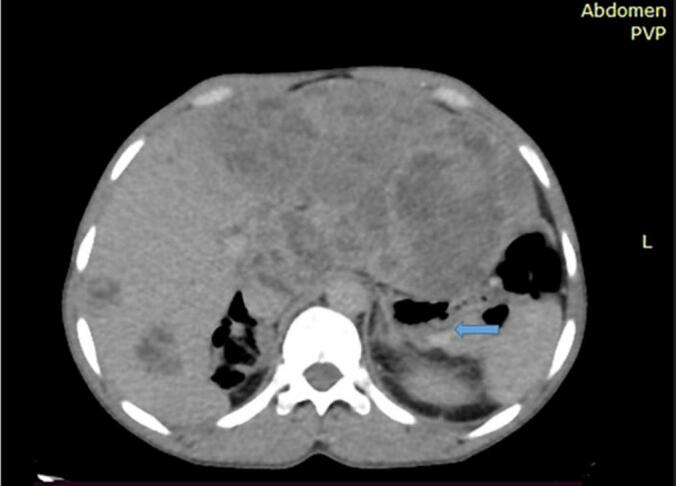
Ultrasound guided FNAC of the gastric mass and from the liver showed epitheloid GIST with secondary liver deposits.
